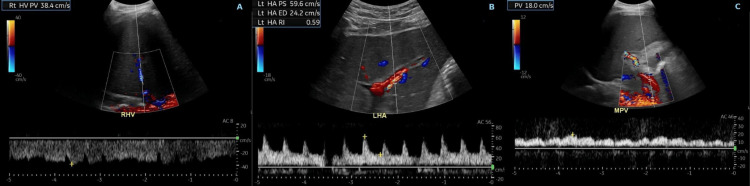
Liver ultrasound of the patient. Rt: right; HV: hepatic vein; PV: portal vein; Lt: left; HA: hepatic artery; PS: peak systolic velocity; ED: end diastolic velocity; RI: resistive index
